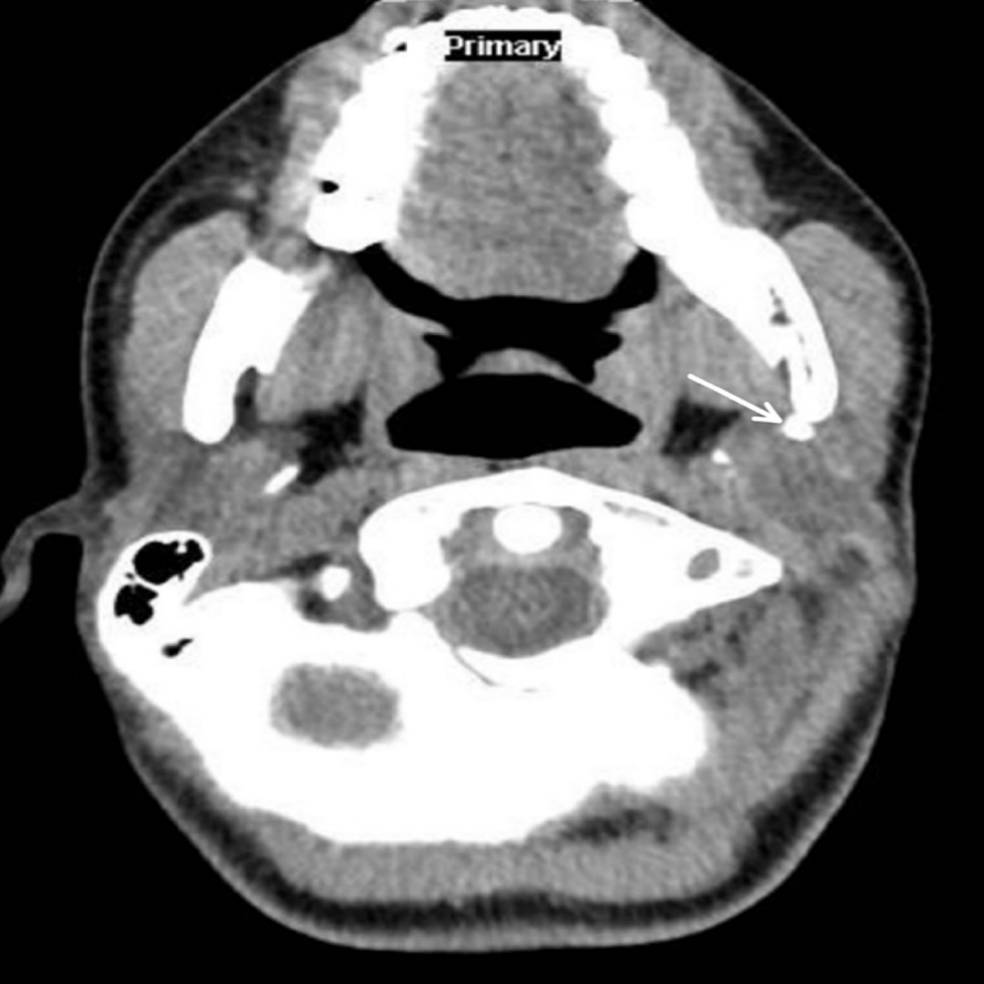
Axial computed tomography image showing the position of the salivary stone, measuring 5 mm, in the deep lobe
